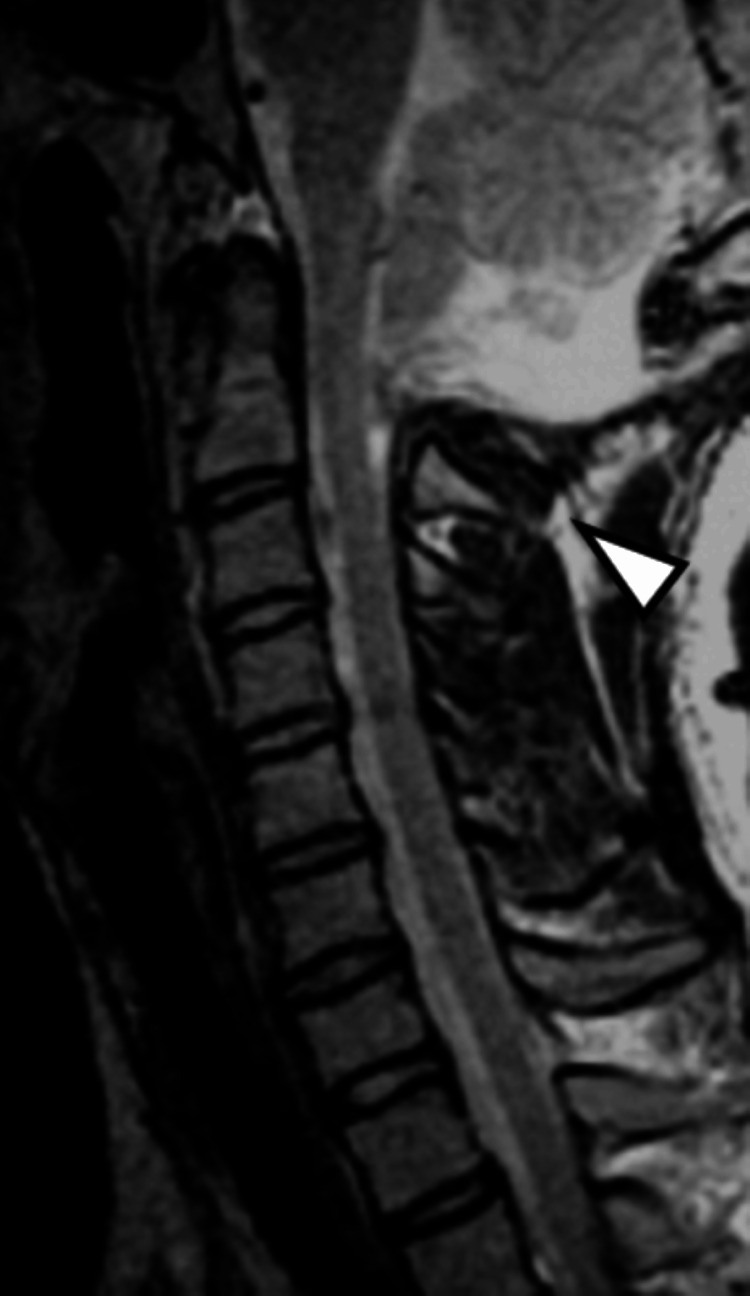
Post-foramen magnum decompression MRI (T2-weighted sagittal view). Foramen magnum decompression, C1 laminectomy, and duraplasty were performed for Chiari malformation type I. Cerebellar tonsillar herniation has improved (arrowhead).
